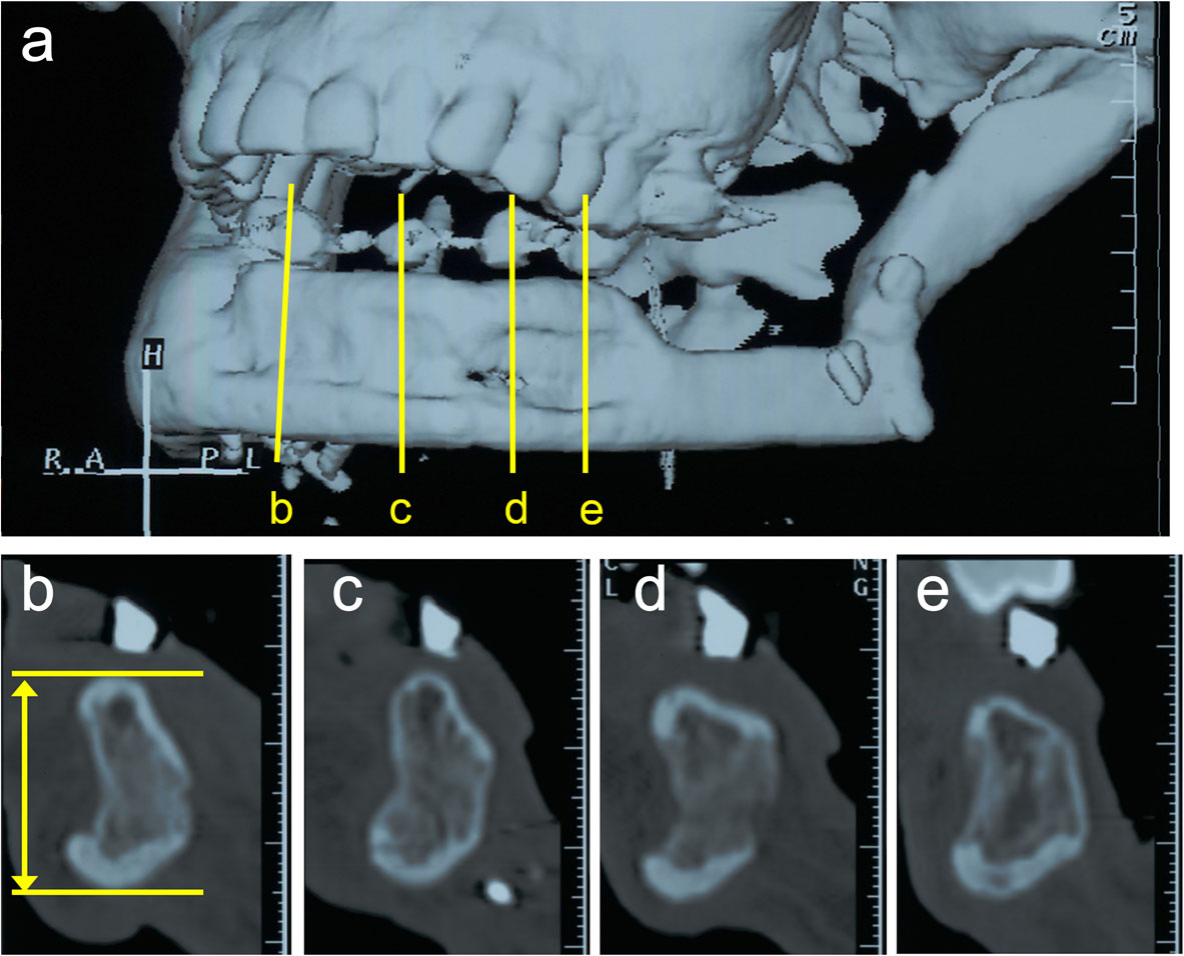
a 3-D computed tomography scan 7 months after vertical distraction osteogenesis of the free revascularized fibula flap. b–e Cross-sectional images of 7 months after vertical distraction. The height of the distracted fibula was approximately 23 mm
